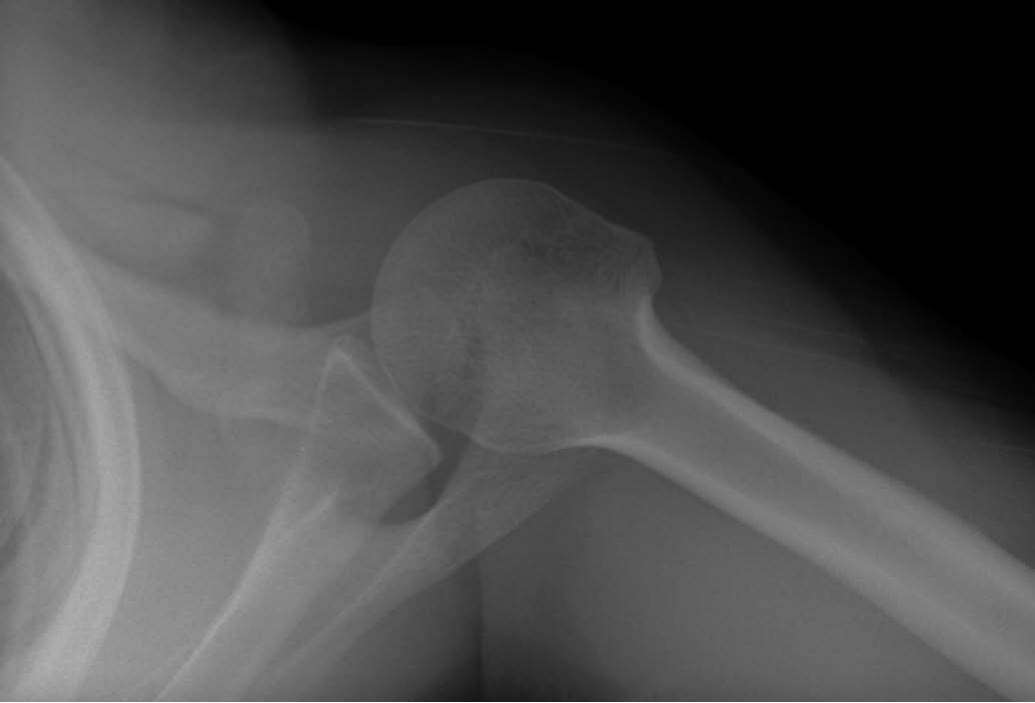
Axillary radiograph showing the pathologic coracoid fracture.
